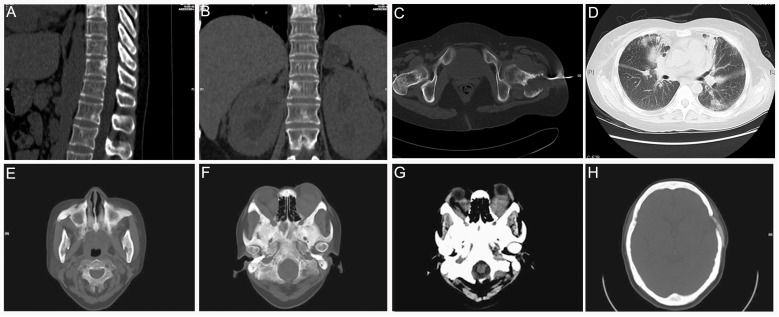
Patient 6: (A,B) Vertebrae involvement was detected on sagittal and coronal multiplanar reconstruction CT. (B) Enlargement and nephrydrosis of bilateral kidneys were also observed. (C) Axial CT shows biopsy of the left proximal femur. (D) Axial CT shows progressive pulmonary interstitial fibrosis. (E,F) Axial CT scans showed bilateral osteosclerosis encompassing the sphenoid sinuses. (G) Exophthalmos caused by bilateral retro-ocular intraconal infiltration. (H) Corresponding axial CT shows biopsy of the left temporal bone.
